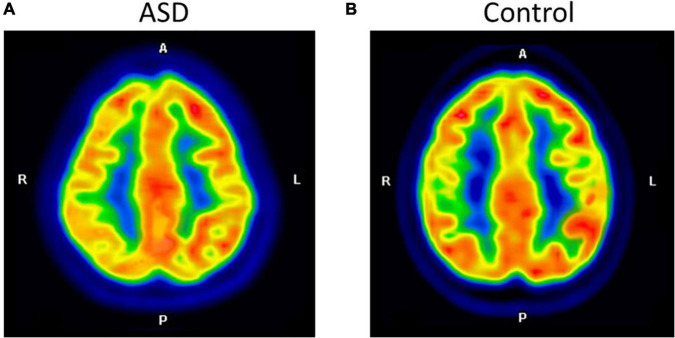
The axial images of the F-18 FDG PET/CT scan demonstrated relatively decreased FDG uptake at the right parietal inferior compared to the left parietal inferior in ASD (A). The control participant shows normal FDG uptake in the same region (B).
